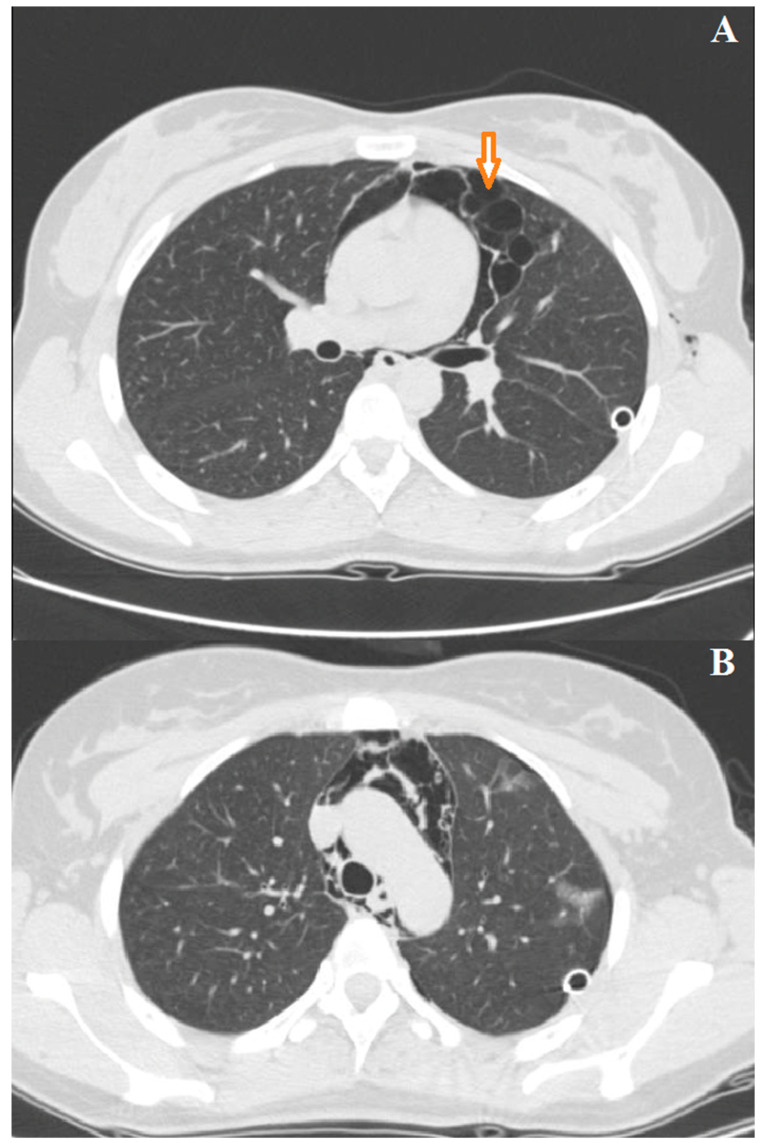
(A,B) Chest CT scan at resolution of left re-expansion edema; the arrow indicates bullous emphysema.
